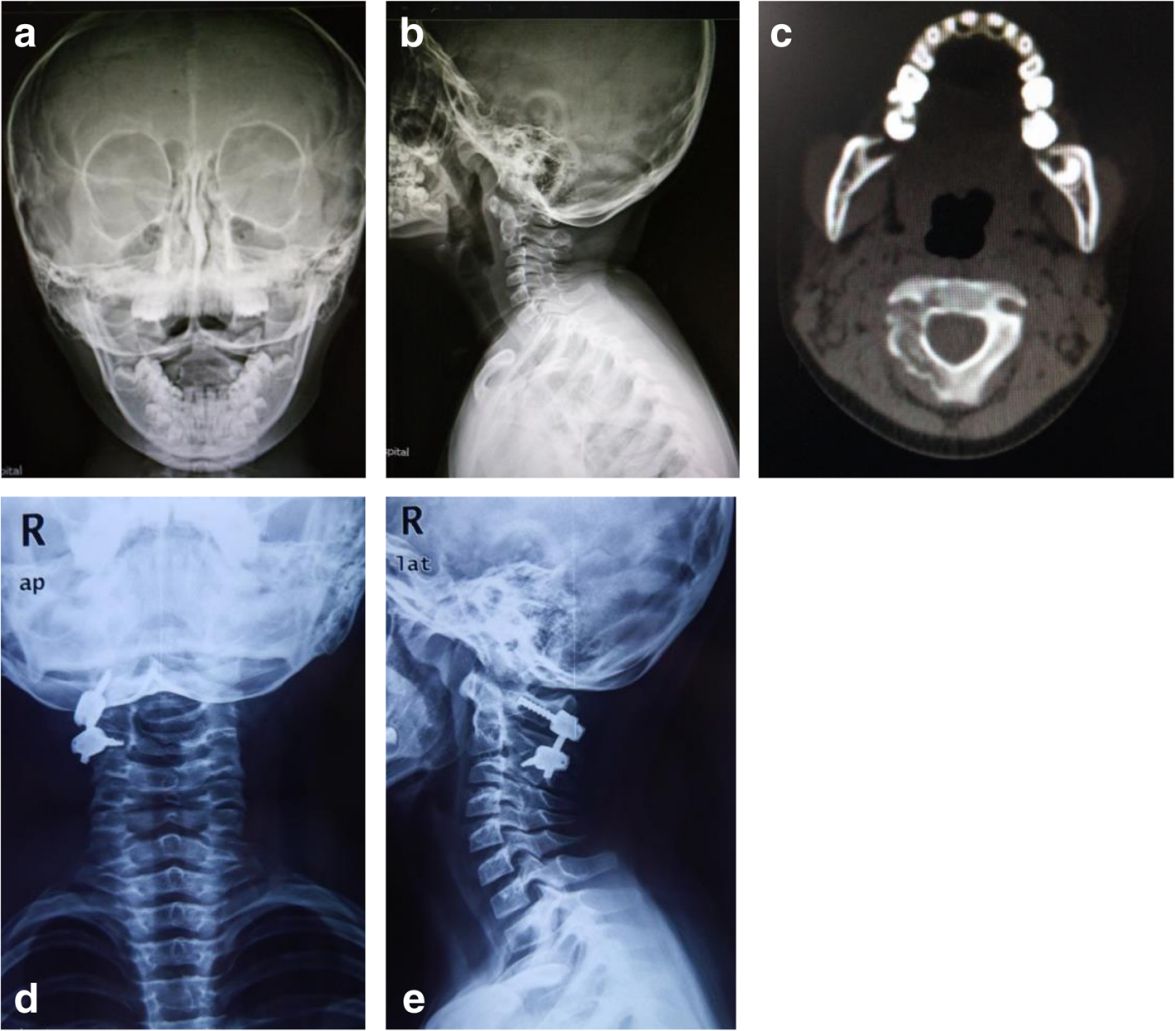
Case 10: a 5-year-old girl, C2 LCH. a, b Anterioposterior and lateral plain film before operation. c CT scan showed the right appendix of C2 was involved. d, e Anterioposterior and lateral plain film 48 months after operation of anterior tumor resection combined with posterior pedicle screw instrumentation
